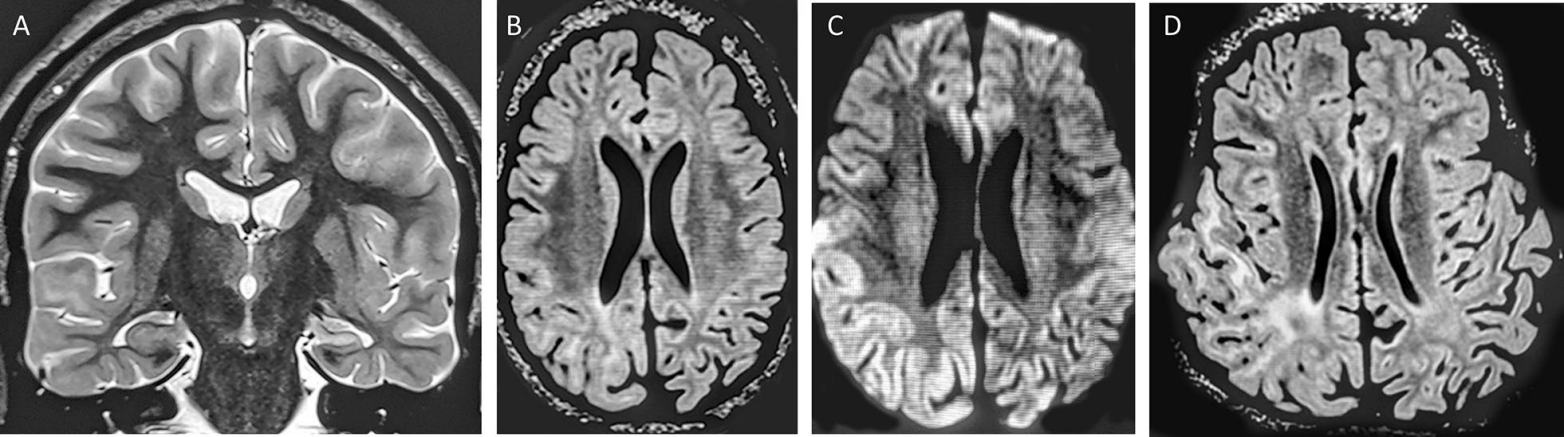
Etiology of Lennox–Gastaut syndrome was assumed due to various structural pathologies. Coronal PD-weighted image shows hippocampal sclerosis and atrophy of the temporal lobe, whereas the right superior temporal gyrus is swollen and white matter is hyperintense (A). The axial FLAIR (B) and corresponding DWI (C) show ictal swelling of the right parietal gyri with white matter edema and cortical diffusion restriction, while the contralateral gyri in the left hemisphere are atrophic. Coronal surface reformatting of the 3D FLAIR (D) shows how the ictal changes extend across the temporoparietal brain region. See again atrophy in the left hemisphere
